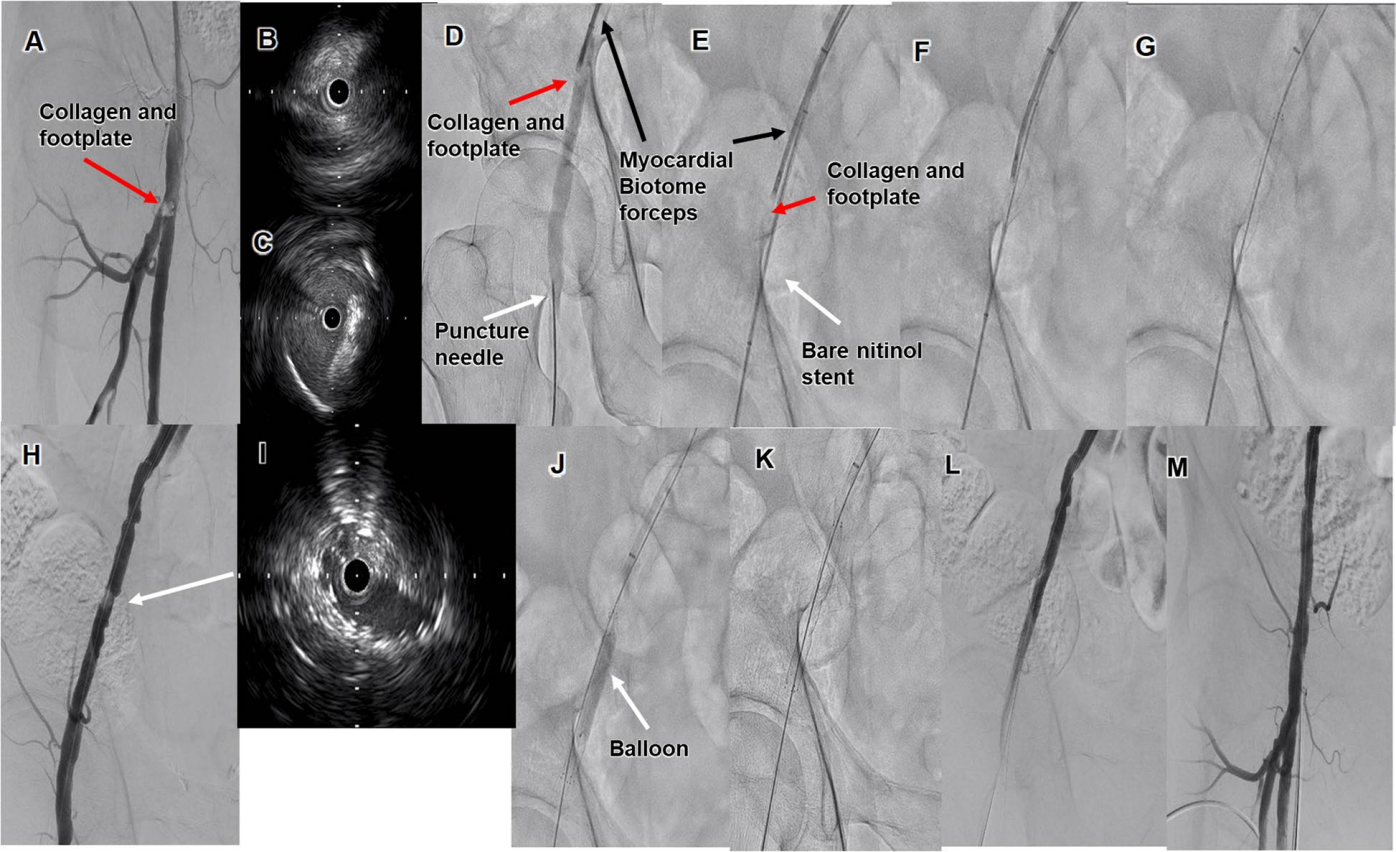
Angiogram of Case 1 after the Angio-Seal drop. A The Angio-Seal collagen stopped at the bifurcation of the deep femoral artery (DFA). B Intravascular ultrasound (IVUS) observation from the DFA shows that the Angio-Seal collagen occupies the DFA, and the DFA is almost occluded. C IVUS observation from the superficial femoral artery (SFA) shows that the Angio-Seal collagen is in the SFA but is not occluding the SFA. D A biotome grasps the Angio-Seal collagen and pulls it up to the external iliac artery (EIA). E-F While the biotome forceps grasp the collagen, a 10.0- × 80-mm stent (SMART^®^; Cordis, USA) is deployed to the EIA and the Angio-Seal collagen is positioned between the stent and the vessel wall. G After stent deployment, the stent expansion is not completed. H Angiography performed after stent deployment shows that the collagen-inserted stent portion is not fully dilated. I IVUS shows that the Angio-Seal collagen is pushing the stent. J Post-dilation of the stent. K-L Angiography performed after post-dilation shows that the stent is fully expanded
